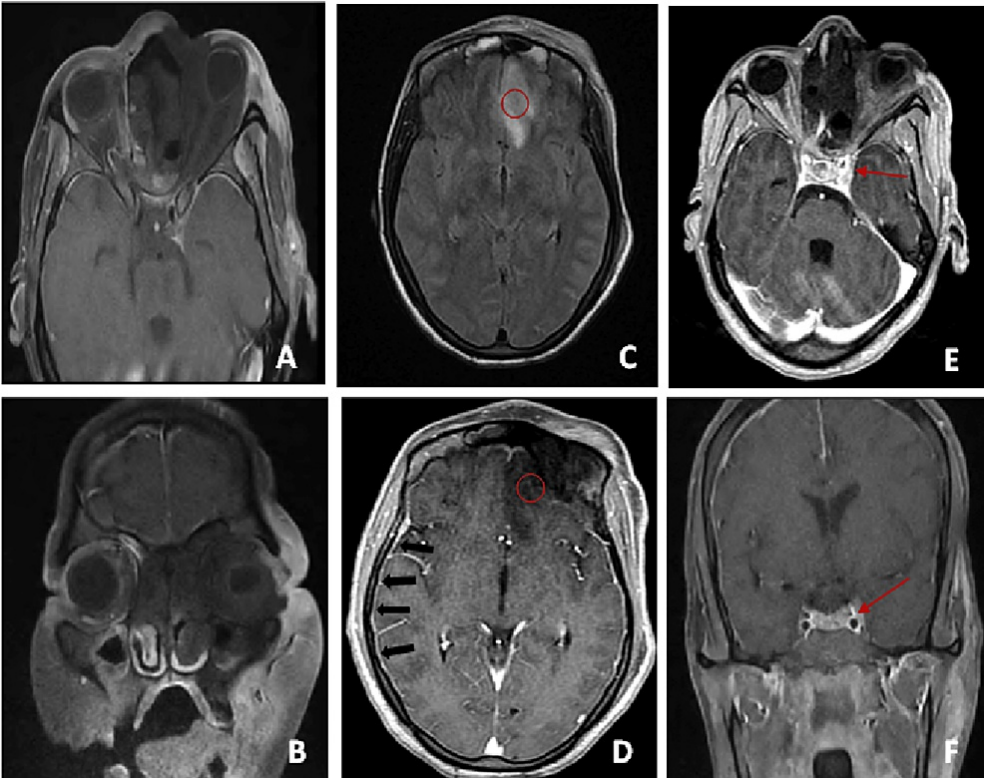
Enhanced T1W axial (A) and coronal (B) MRI sequences showing the black turbinate: lack of enhancement on postcontrast related to frontal, ethmoidal, sphenoidal, and maxillary sinuses necrosis with left orbital extension complicated by grade II left proptosis. Axial brain FLAIR MRI (C) showing a frontal lobe hyperintensity with no T1W contrast-enhancement (D) (circle) associated with meningeal enhancement (black arrows) due to meningoencephalitis. Enhanced three-dimensional T1W axial (E) and coronal (F) sequences showing enlargement of the left cavernous sinus with lack of T1W contrast-enhancement related to sinus thrombosis (red arrows). T1W: T1-weighted; FLAIR: fluid-attenuated inversion recovery.
